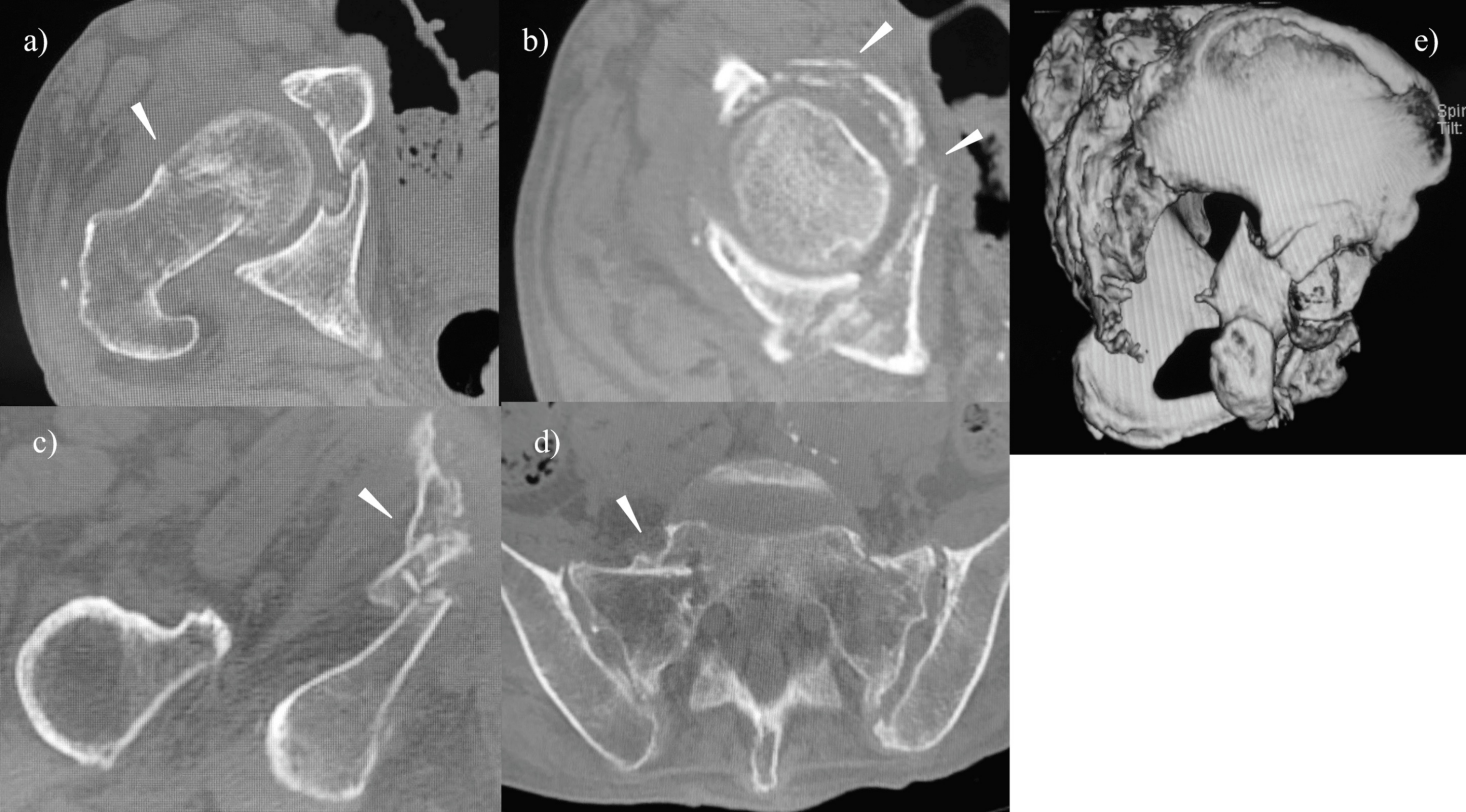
Preoperative CT of case 1 The white arrow indicates a) femoral neck fracture, b) acetabular fracture, c) pubic ramus fracture, and d) sacral ala fracture, and e) a 3D CT scan posterior column fracture was identified
